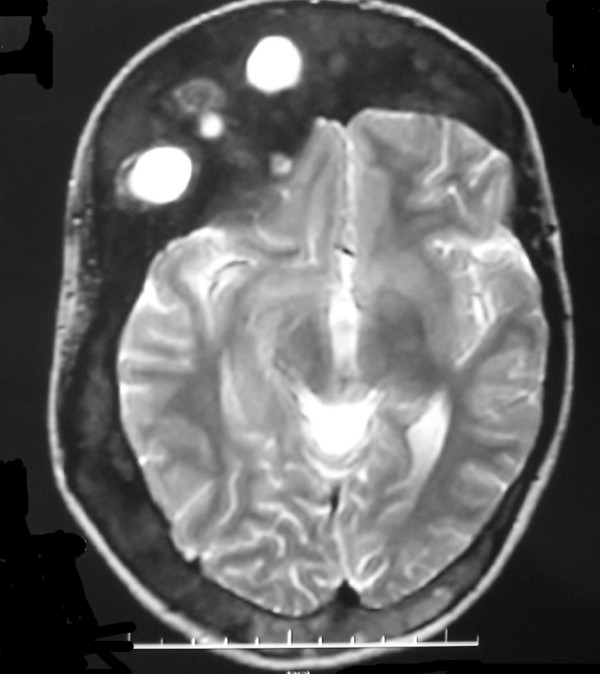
T2-weighted MR image show cysts of frontal bone manifest as hypertensity with well-defined. These lesions are more frequent in the craniofacial bones.
